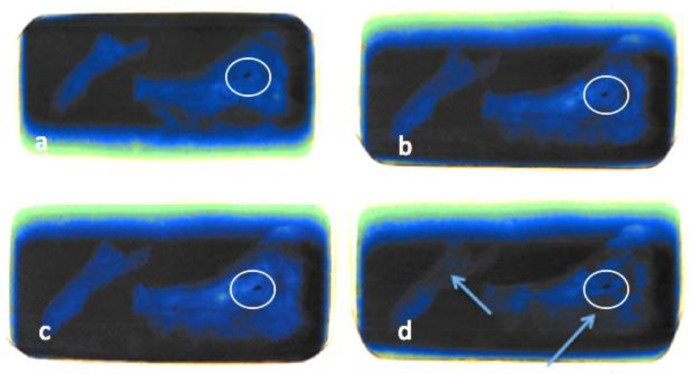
(a–d) Comparisons of X-ray images from 30 September 2020 (a), 21 December 2020 (b), 3 March 2021 (c) and 25 August 2021 (d). The darkening is shown by arrows. The projectile is marked with a circle in all four images. Quality of the images is due to the reduced radiation density in the portable X-ray machine.
